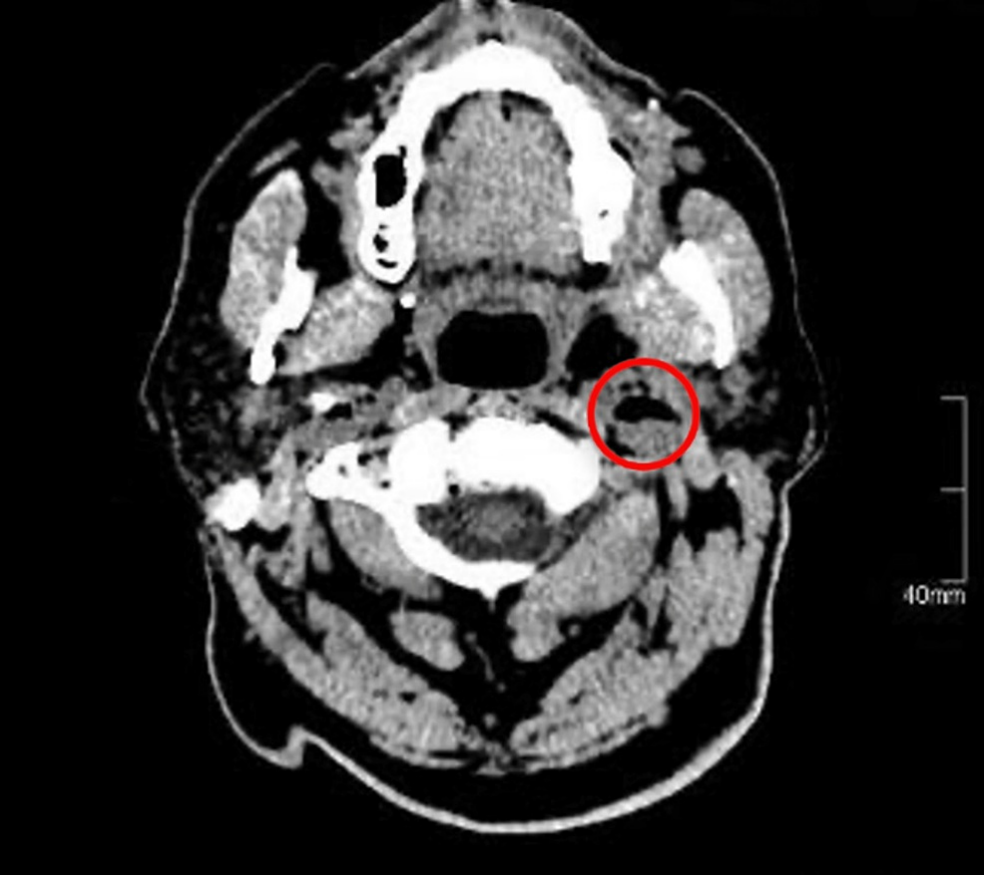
CT imaging of the brain suggests air densities noted within both internal jugular veins, more strongly apparent at the left side.
